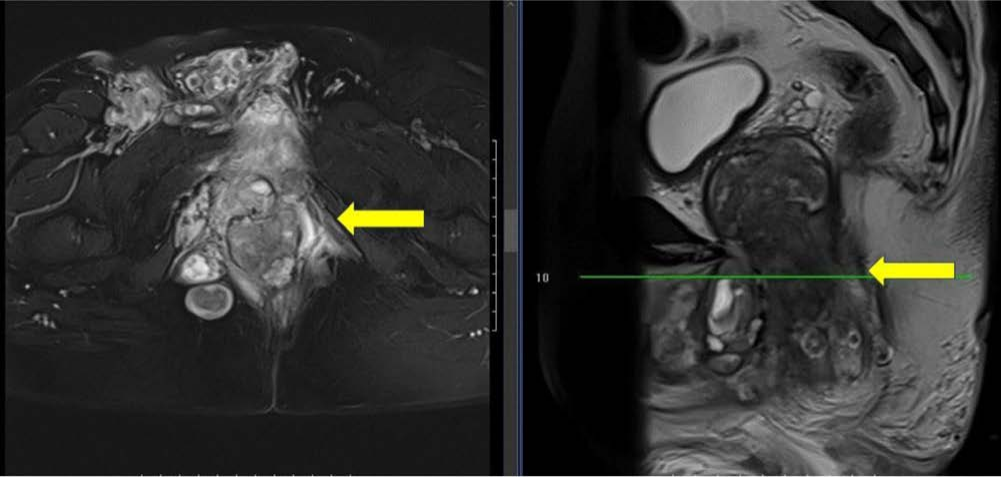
Pelvic MRI of Case 2. MRI = magnetic resonance imaging.
